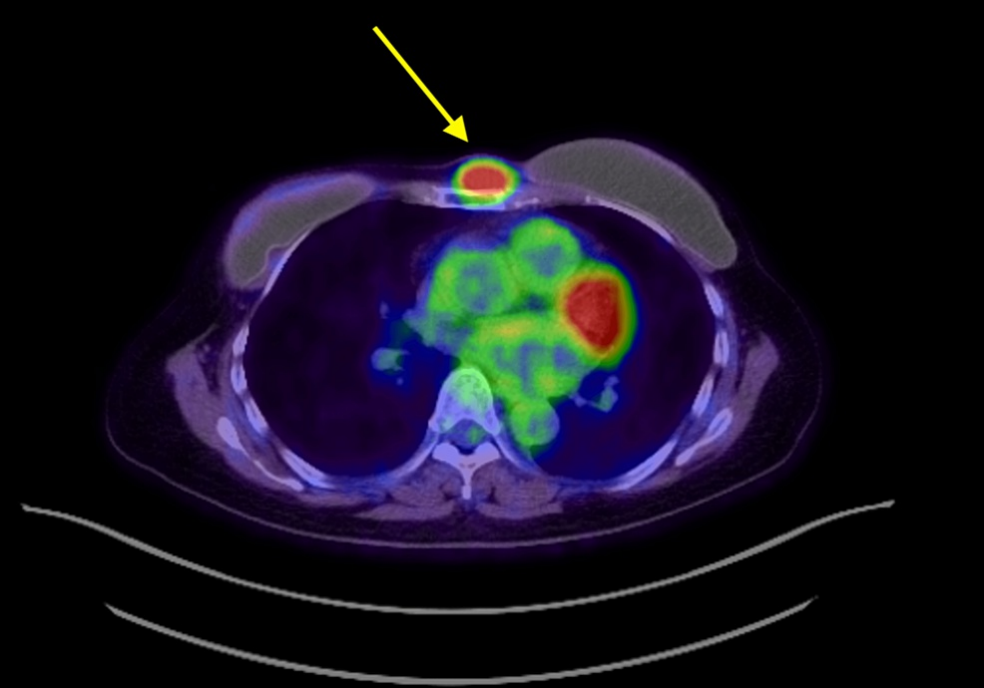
A positron emission tomography-computer tomography of left invasive ductal carcinoma recurrence in the soft tissue of the sternum The patient’s positron emission tomography-computed tomography (PET-CT) image demonstrates a hypermetabolic subcutaneous 2.37 cm x 1.16 cm PET avid nodule (yellow arrow) in the soft tissue of the anterior chest left to midline.
